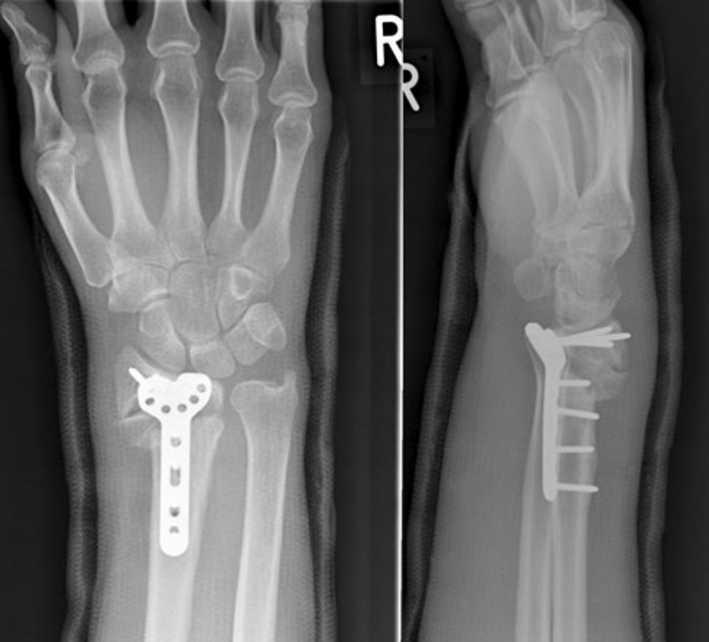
Radiographs at 6 weeks after the second revision procedure (with bone graft)
